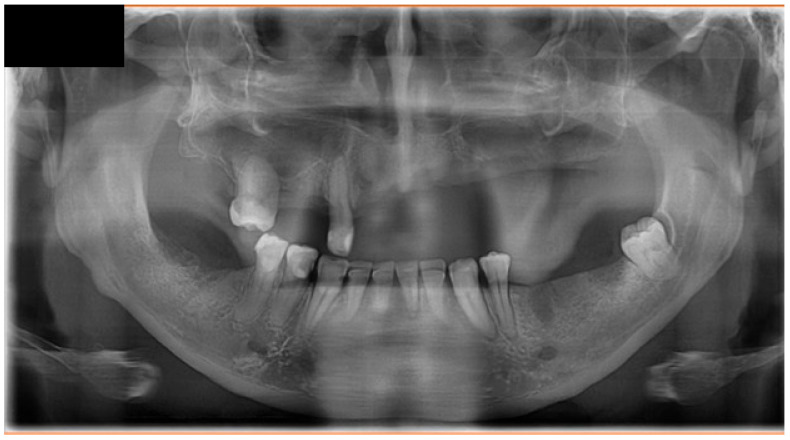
Digital orthopantomogram (DOP) obtained during clinical remission, demonstrating stabilization of maxillary structures following combined oncologic treatment and supportive dental management.
